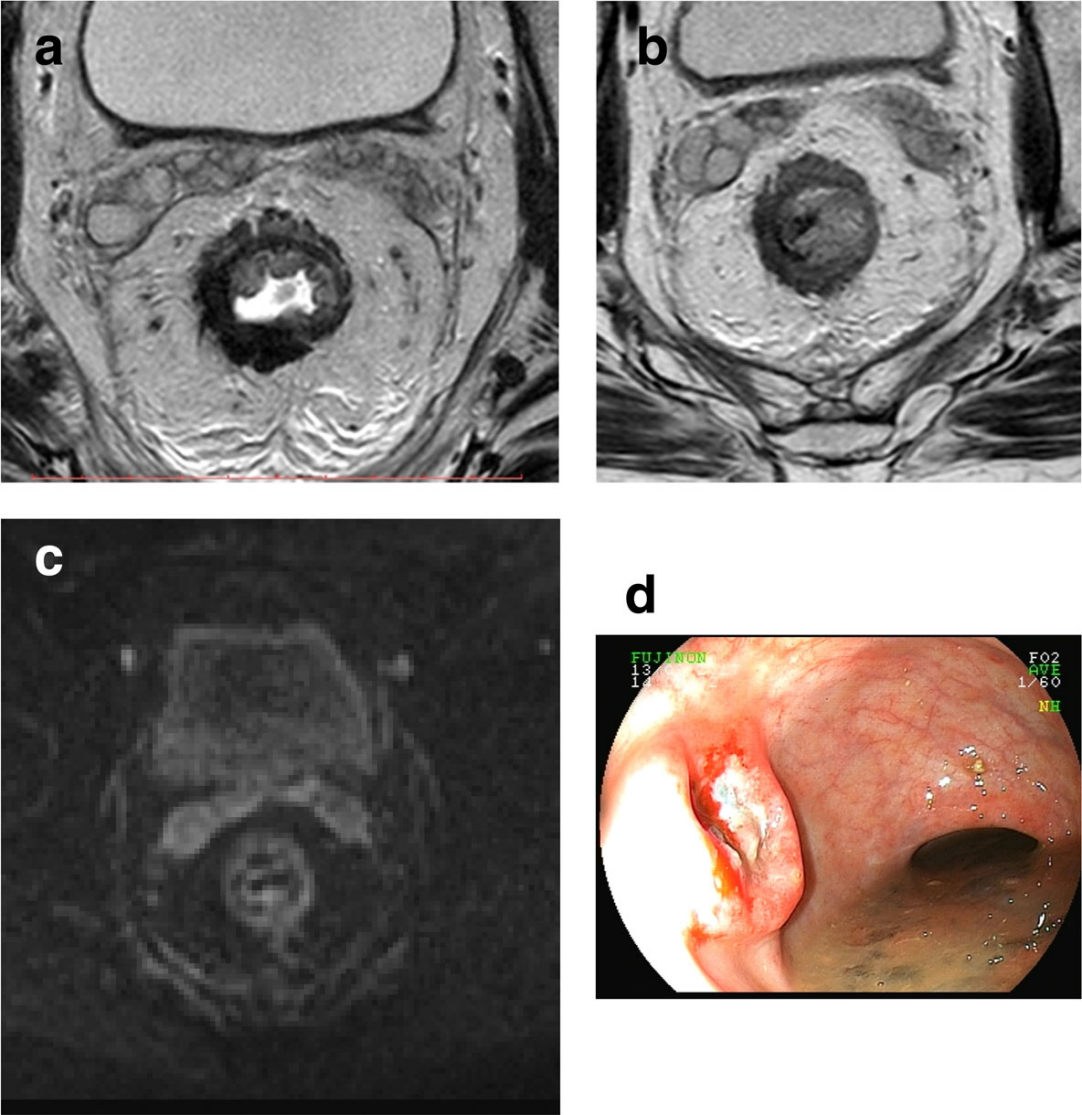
Luminal regrowth detected exclusively by endoscopy. Images show a clear endoscopic view of a regrowth following initial complete clinical response with no relevant radiological findings. a High-resolution T2WI restaging axial image 16 weeks after neoadjuvant therapy shows a low signal intensity scar from 5 to 9 o’clock. Watch-and-wait was offered. b Follow-up exam after 12 months showed less extensive, from 7 to 10 o’clock persistent low signal intensity scar with no signs of regrowth. c DWI (*B* = 700) was unremarkable. d Endoscopy showed obvious regrowth at 12 months and the patient was sent to salvage resection. Total mesorectal excision was performed; ypT2N0 with free margins was the final result
